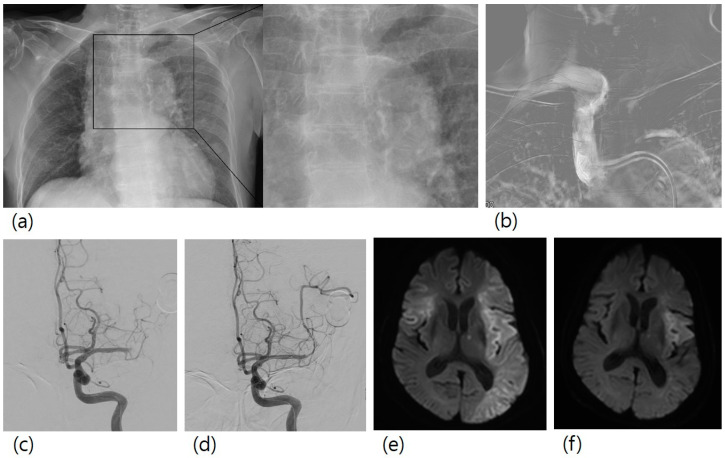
A case of mechanical thrombectomy for left M1 occlusion. A 78-year-old female patient with right hemiparesis visited our hospital. (a) Her chest X-ray showed aortic arch calcification. (b) In the first step of the procedure, guiding catheter was advanced to the brachiocephalic artery, and a roadmap image was obtained. (c) Left M1 occlusion was observed. (d) Mechanical thrombectomy with stent retriever was conducted, and successful recanalization was achieved. (e) Diffusion-weighted imaging two days after mechanical thrombectomy showed a new lesion in the right MCA territory, (f) which was not observed in diffusion-weighted imaging before mechanical thrombectomy.
